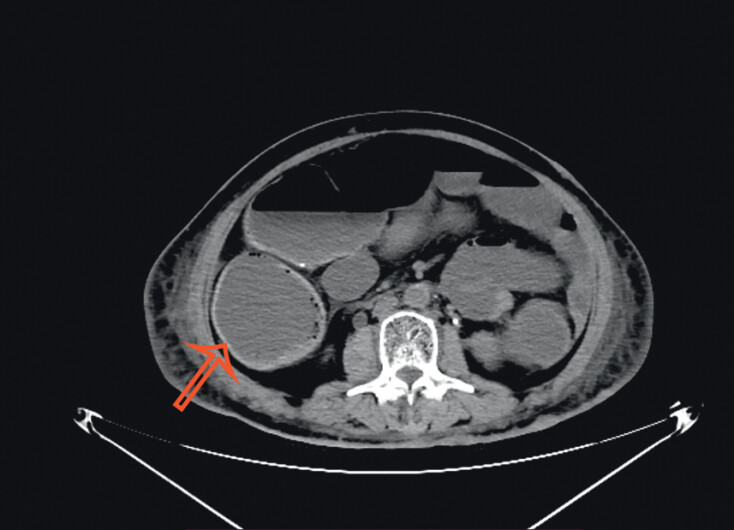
Preoperative abdominal CT showing markedly dilated bowel loops
